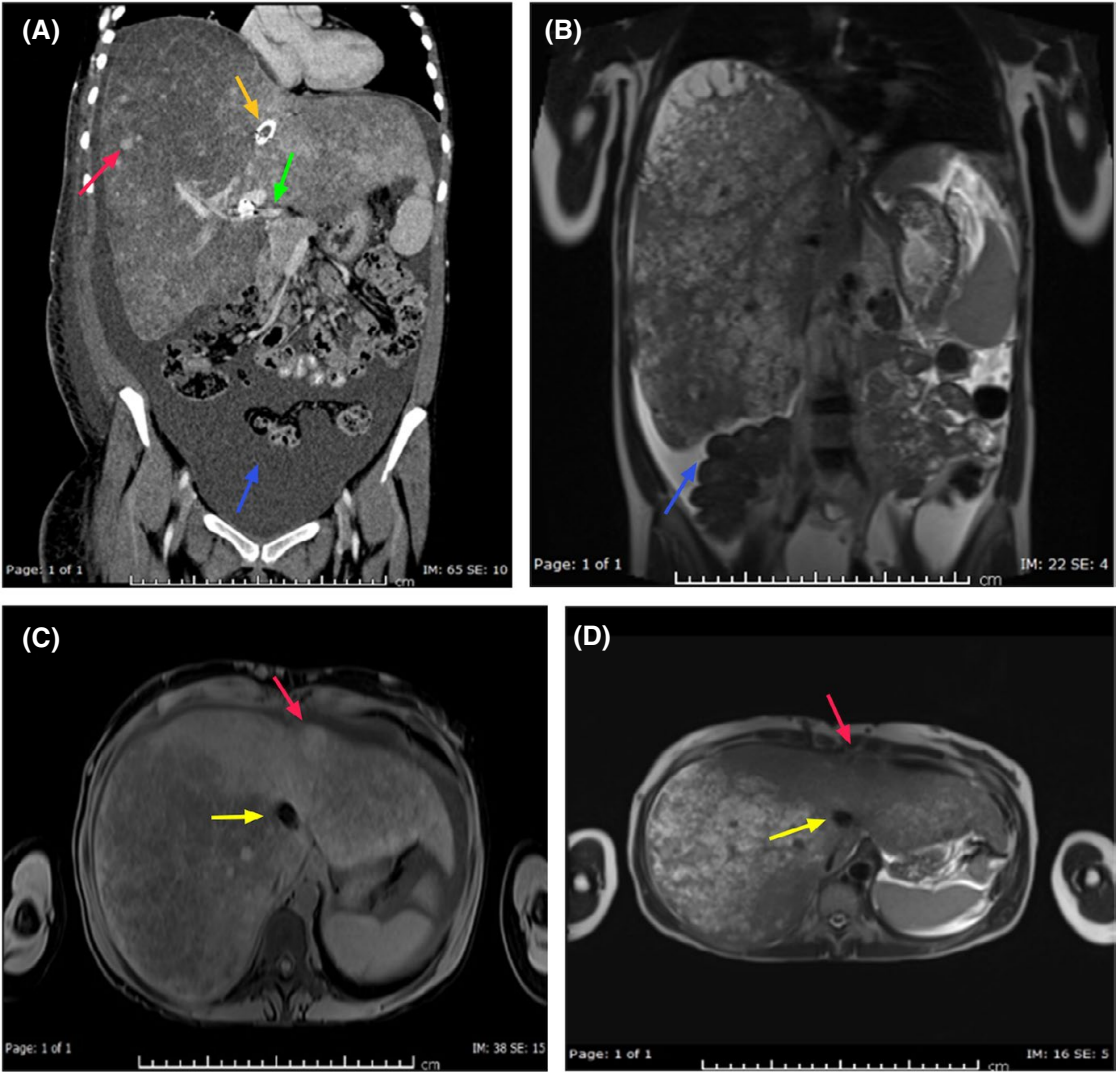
(A) Coronal CT scan of the abdomen with oral and intravenous contrast showing markedly enlarged liver with the span of the right lobe 28 cm, multiple liver nodules (red arrow), moderate ascites (blue arrow), patent portal vein (green arrow) and Transjugular intrahepatic portosystemic shunt (TIPSS) (yellow arrow). (B) Coronal MRI of the abdomen T2 WI. (C) Axial T1 WI of the abdomen. (D) Axial T2 WI of the abdomen showing enlarged heterogeneous liver with multiple nodules (red arrow), ascites (blue arrow), and TIPSS (yellow arrow)
